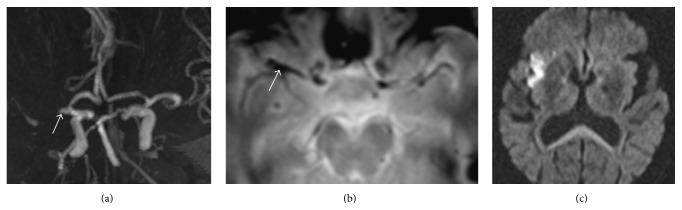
The MCA susceptibility sign in LAA patients. (a) MRA reveals an MCA occlusion. (b) T2^*∗*^-weighted MR imaging indicates a low-signal image with a relatively small diameter and long length along the MCA. (c) DWI shows that the infarct size was relatively small compared to CE patients.
